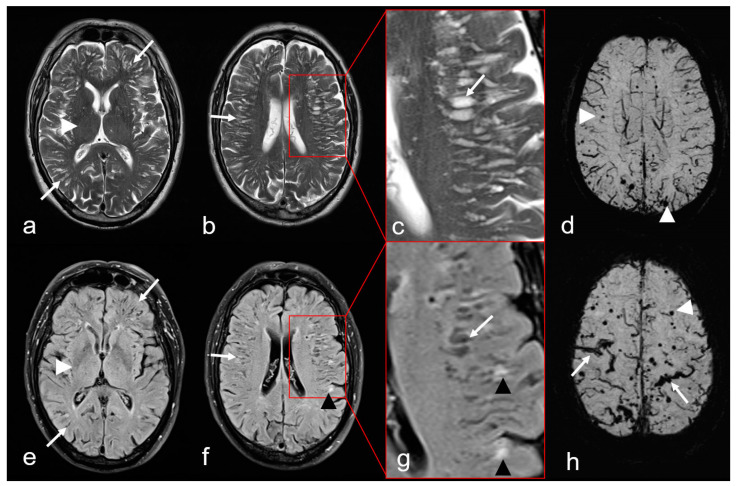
Non-hemorrhagic and hemorrhagic MRI features in cerebral amyloid angiopathy (CAA). Severe enlarged perivascular spaces (PVS) supratentorial ((a–c): T2-weighted images (WI), arrow); (e–g): Fluid attenuated inversion recovery (FLAIR) images (arrow) sparing the basal ganglia ((a,e): white arrowhead), characteristic for a centrum semiovale (CSO) PVS pattern. (f,g): Multiple partially conflating white matter hyperintensities in a multispot pattern (black arrowhead, WMH-MS). (d,h): Susceptibility-weighted images (SWI) exhibit additional hemorrhagic lesions, i.e., multiple cortical/subcortical microbleeds (MB, white arrowhead) and multifocal cortical superficial siderosis (cSS; (h), arrow); MRI 1.5 T Intera, Philips Healthcare.
